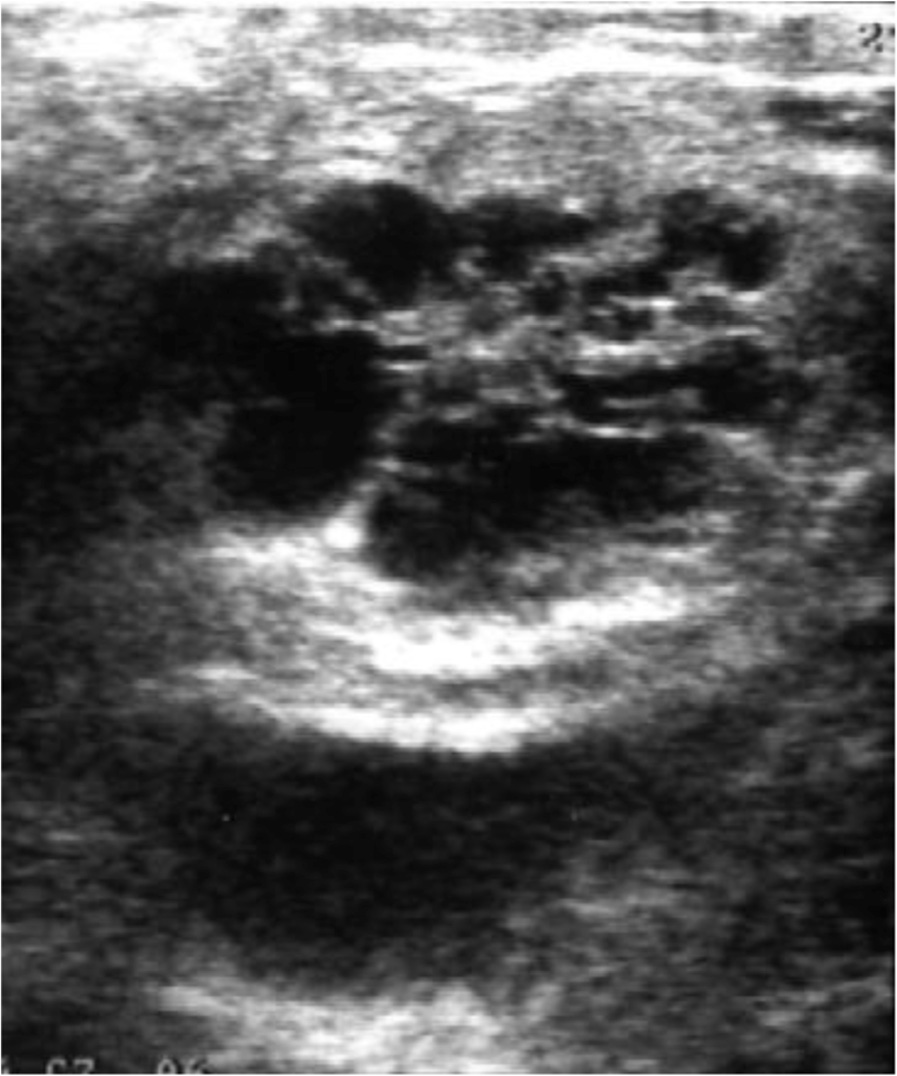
Intraoperative ultrasound examination. A well-demarcated honeycomb-like cystic lesion in the pancreatic uncus was found. There was no evidence of venous or ductal invasion
